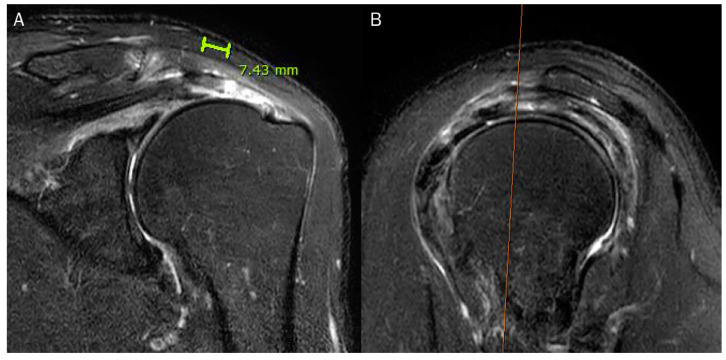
Arthroscopically proven full-thickness tear of the supraspinatus tendon without delamination. (A) Oblique coronal fat-suppressed T2-weighted image and (B) coronal fat-suppressed T2-weighted image demonstrate the full-thickness tear of the supraspinatus tendon with a visible cleavage measured at least 1 cm in length. The tendon was not classified as a delaminated tear.
